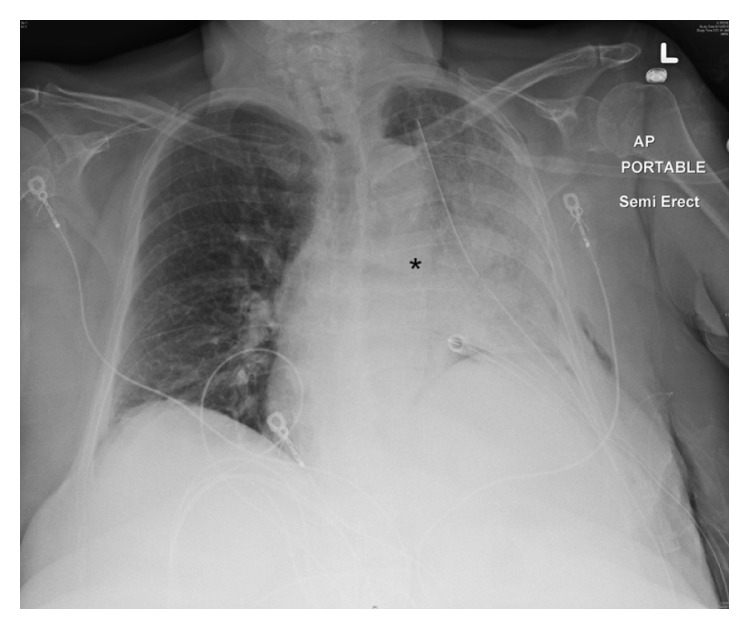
Portable chest radiograph obtained on postop day-2 showing left lung opacification with bronchial cutoff (black asterisk).
